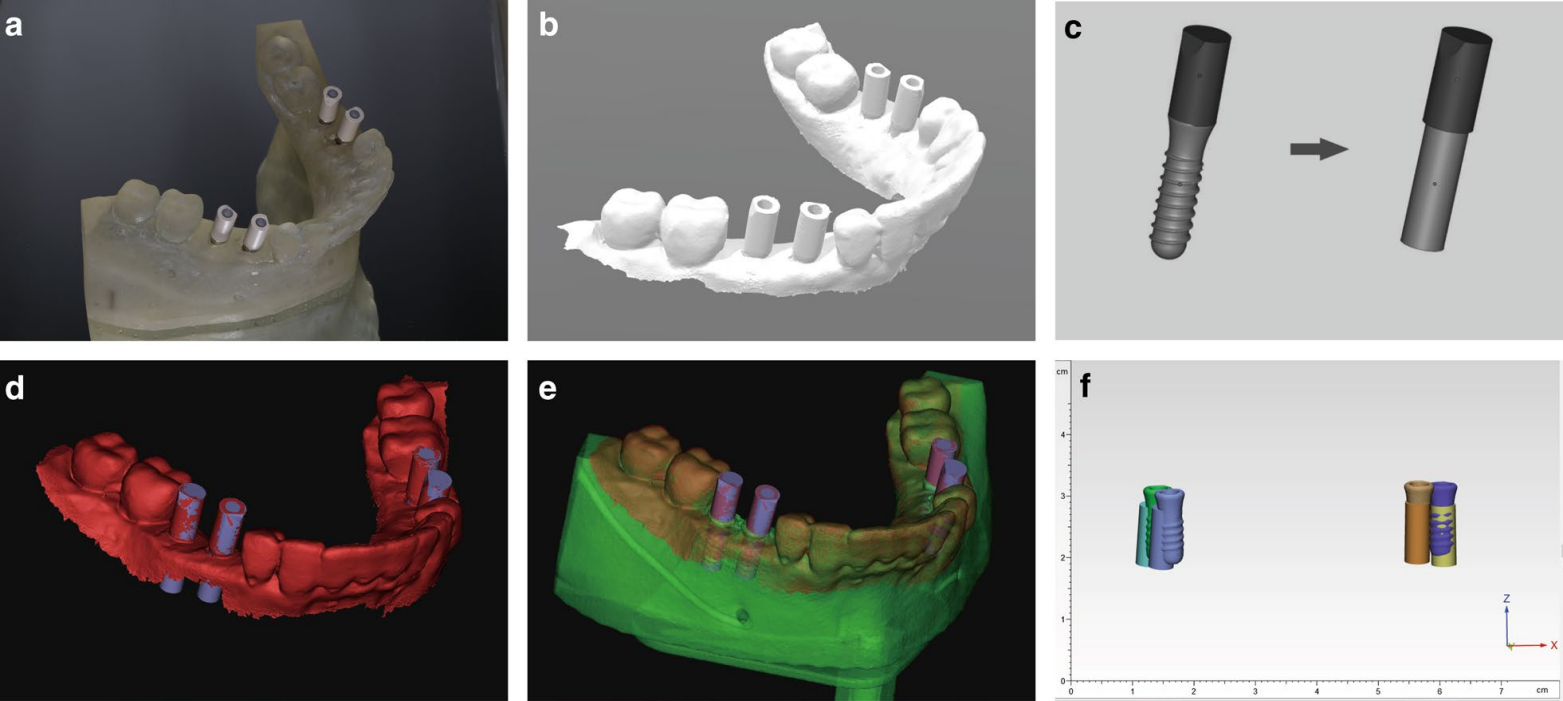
Dental implant location via surface scanner. a Scan bodies were connected to the implants placed in the resin mandible model; b the model was scanned with intraoral and extraoral scanners; c location component: scan body and implant were connected virtually and the implant part was simplified to a cylinder of same diameter and length; d The location component was aligned in the surface scanning image by matching the geometry of scan body; e the resulted model in (d) was aligned in the CBCT images by matching the remaining cusps in the model; f The positions located via surface scanning was compared with the position in CBCT in the software 3-matic
